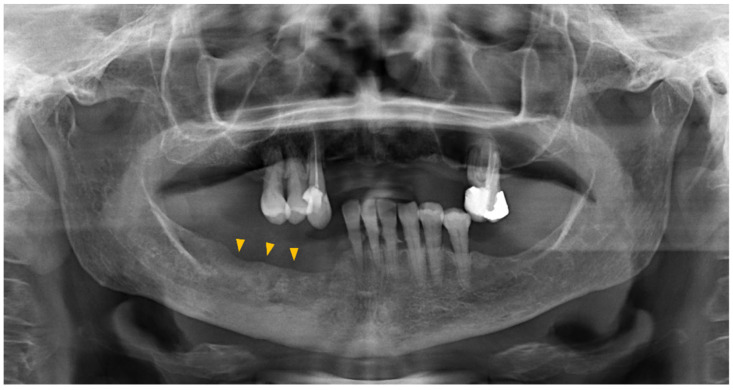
The orthopantomogram of the patient depicted in Figure 2 reveals osteosclerosis in the body of the right mandible, exhibiting minimal indications of bone resorption (indicated by yellow arrows) in comparison to the findings observed in the CT scan. It is worth mentioning that the CT scan also detected a fracture in the inferior border of the mandible, in contrast to what is observed in the orthopantomogram.
